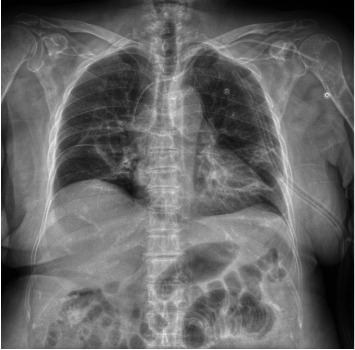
Simple chest X-ray showing adequate resolution of pneumothorax, pneumomediastinum, and decrease of the subcutaneous emphysema.
